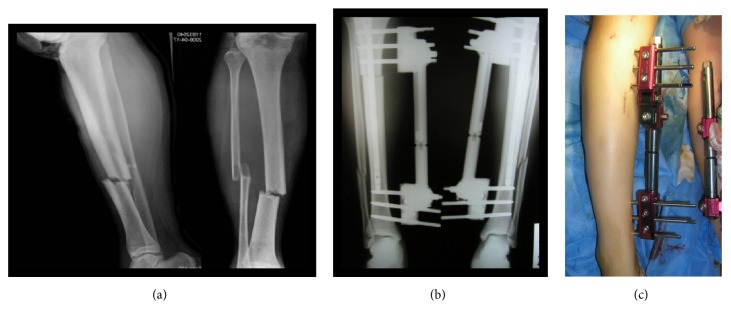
A clinical case. (a) Preoperative X-ray film for a case of tibia deformity. (b) Postoperative anteroposterior X-ray film of limb. (c) Photo showing the limb after application of the unilateral external fixator.
